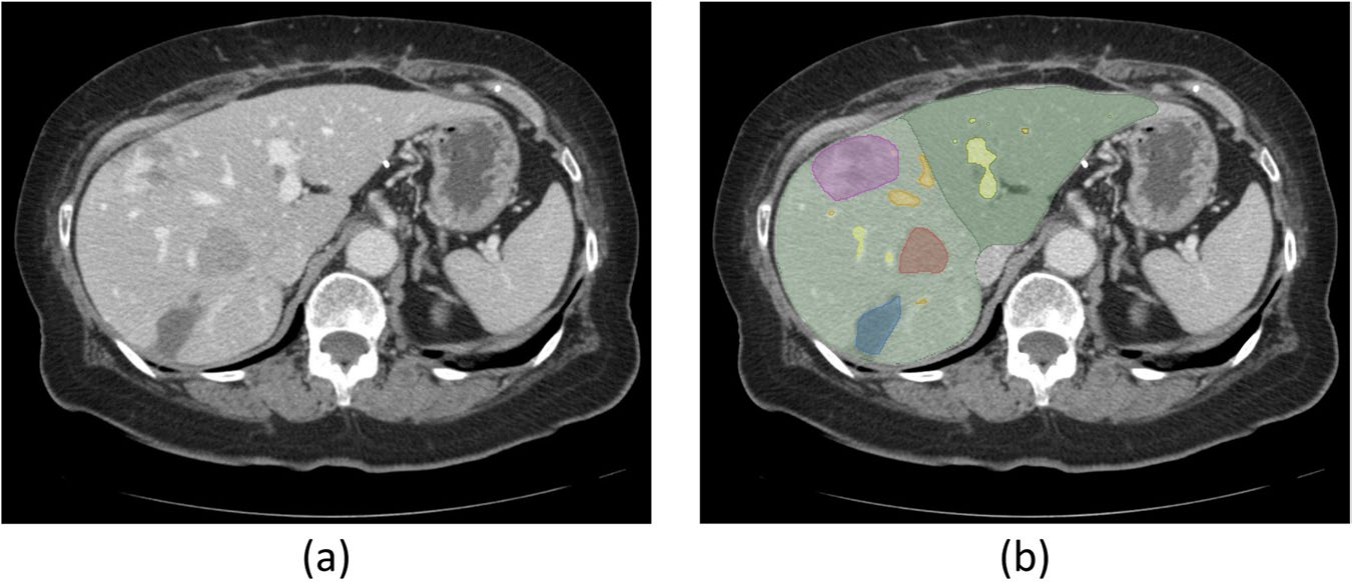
A sample CT image slice with segmentations. (a) A slice of the CT volume. (b) Segmentations of the liver (green), liver remnant (darker green), hepatic and portal veins (orange and yellow), and tumors (red, blue, purple).
